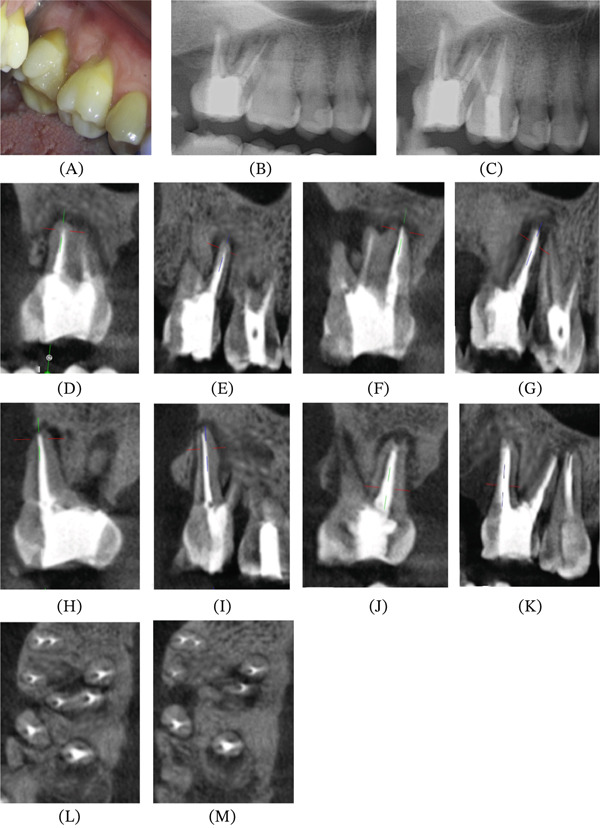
Clinical, radiographic and CBCT follow‐ups. (A) Healing of the mucosal sinus tract in a 12‐month clinical follow‐up. (B) Periapical radiograph after 12 months. (C) Periapical radiograph after 24 months. (D) A 24‐month CBCT coronal section of the MB root. (E) A 24‐month CBCT sagittal section of the MB root. (F) A 24‐month CBCT coronal section of the MP root. (G) A 24‐month CBCT sagittal section of the MP root. (H) A 24‐month CBCT coronal section of parastyle root. (I) A 24‐month CBCT sagittal section of the parastyle root. (J) A 24‐month CBCT coronal section of the P root. (K) A 24‐month CBCT sagittal section of the P root. (L, M) The 24‐month CBCT axial sections in the apical root third.
